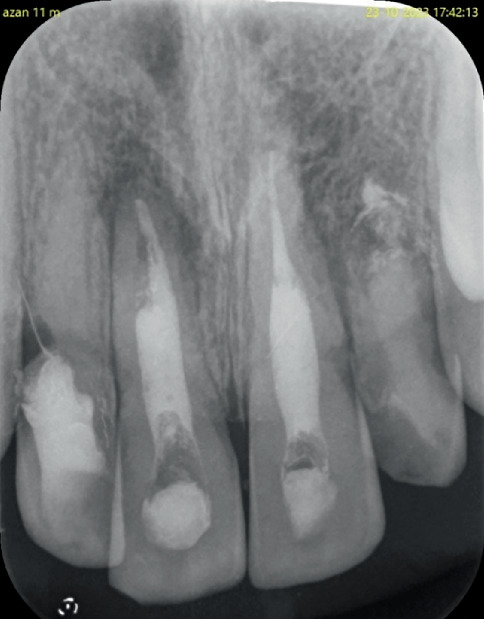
Radiographic image of separated file fragment in cervical defect and obliterated canal space in relation to Tooth Number 12.
